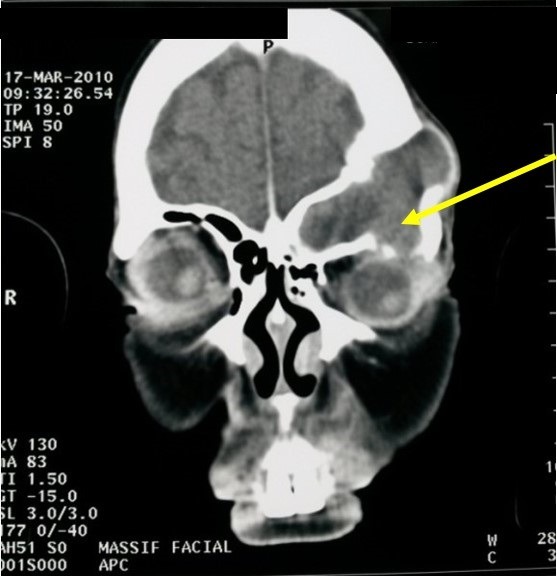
Facial CT scan in frontal section: left frontal mucocele responsible for a lysis in the left roof of the orbit
